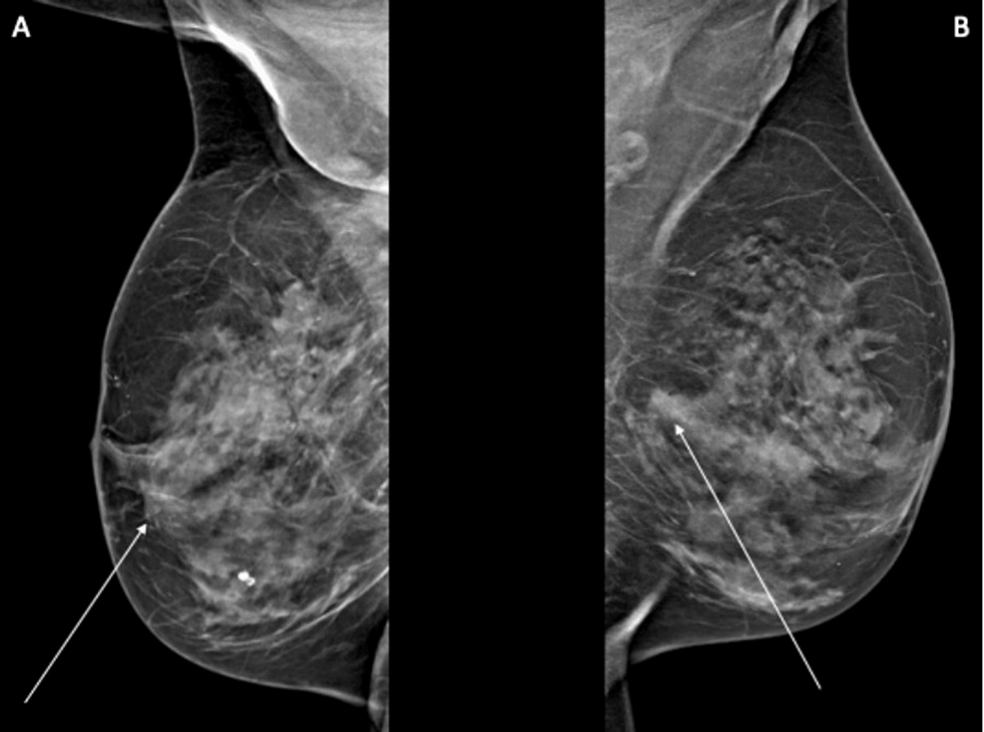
X-rays of both breasts. (A) Right breast: The image shows a periareolar lesion with ill-defined borders measuring approximately 24 × 24 × 24 mm (arrow). (B) Left breast: The image shows a lesion located deep in the breast, prepectoral, with ill-defined borders measuring approximately 10.6 × 5.4 × 6.8 mm (arrow).
